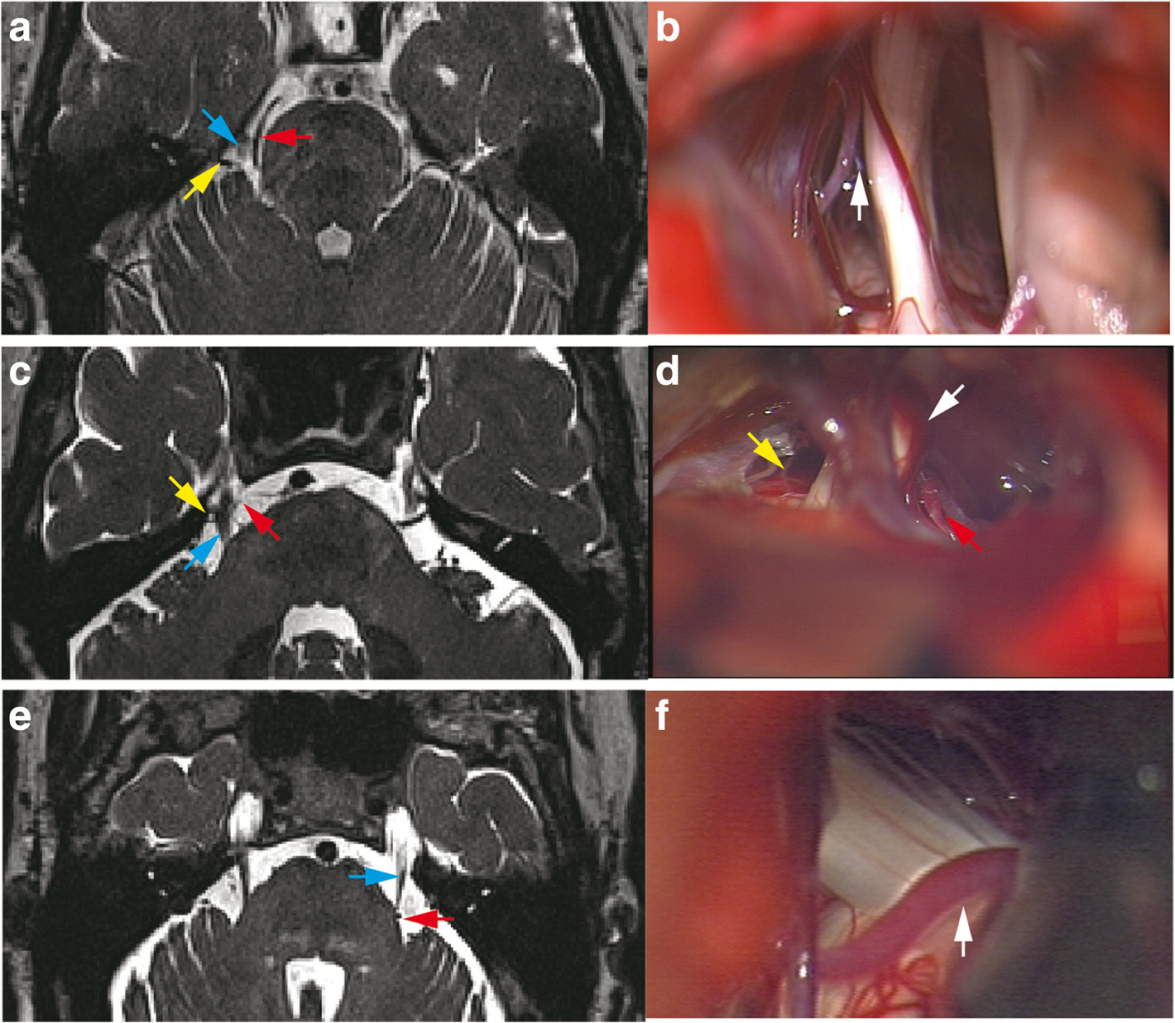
Routine MRI displaying NVC may have different intra-operative findings. a The superior cerebellar artery (SCA, red arrow) and a vessel (yellow arrow) located at the lateral of TGN (blue arrow) were the suspected vessels of NVC. b Intra-operative image showed SCA was close to but not in touch with TGN. A trivial vessel (vein) crawled on TGN. The white arrow points to the gap of the SCA and TGN. c MRI revealed the SCA (red arrow) and another vessel (yellow arrow) each formed a loop conflicting TGN (blue arrow) from inner side and outside. d Intra-operative image showed both vessels did not compress TGN (correspondingly marked with red and yellow arrow). A vein (white arrow) compressed the TGN from lower, outside at REZ to the TGN surface. e A very tiny vessel was displayed by pre-operative MRI at the outside of REZ of left TGN (blue arrow) which was easily neglected (red arrow). f An artery was found compressing the REZ of left TGN during surgery (white arrow)
